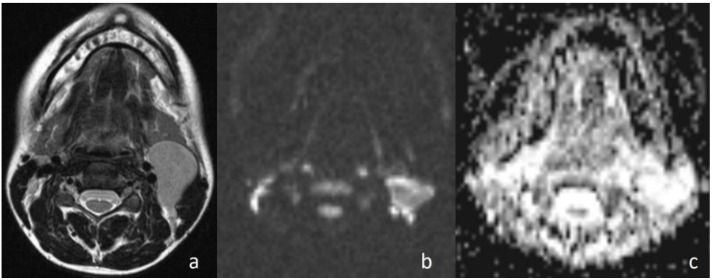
Second branchial cleft cyst. (a) Axial T2WI shows a unilocular cystic mass in the left carotid space; (b) the lesion shows low signal intensity on DWI; and (c) the lesion is hyperintense on the ADC map (ADC value of 2.02 × 10^−3^ mm^2^/s).
